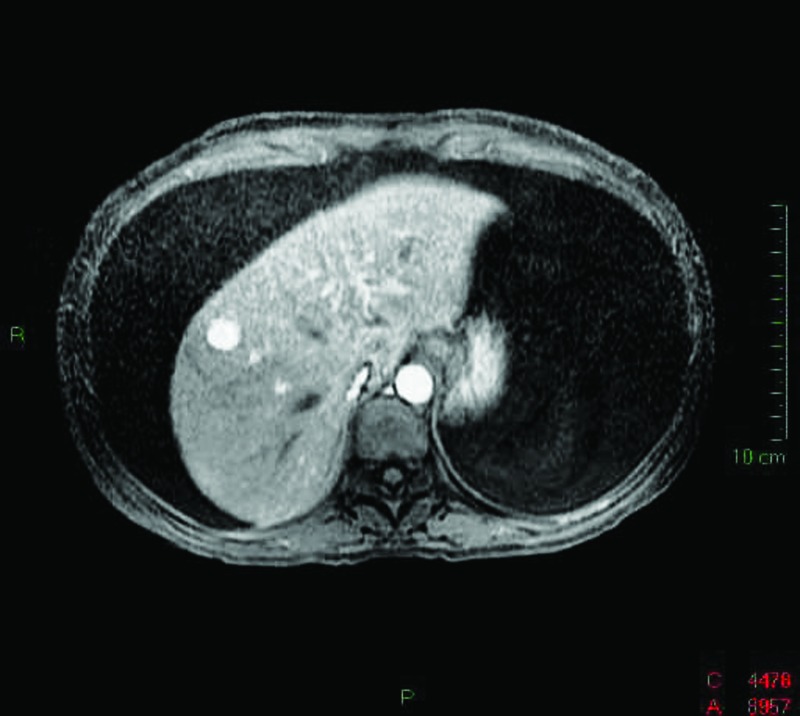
MRI showing massive ascites and a hepatic hamangioma.
